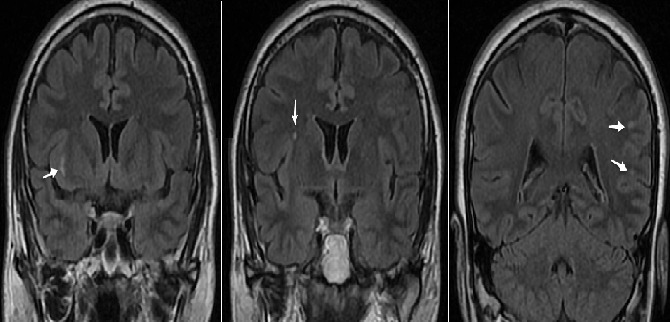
IRM cérébrale en séquence FLAIR chez le 2ème patient: Les coupes coronales montrent quelques lésions nodulaires en hypersignal FLAIR intéressant la substance blanche sous corticale fronto-pariétale bilatérale, temporale et capsulaire externe droite en rapport avec des infarctus lacunaires sous corticaux. La topographie des anomalies de signal prédominant dans les territoires péri-ventriculaires et les centres semi-ovales est très évocatrice du CADASIL
